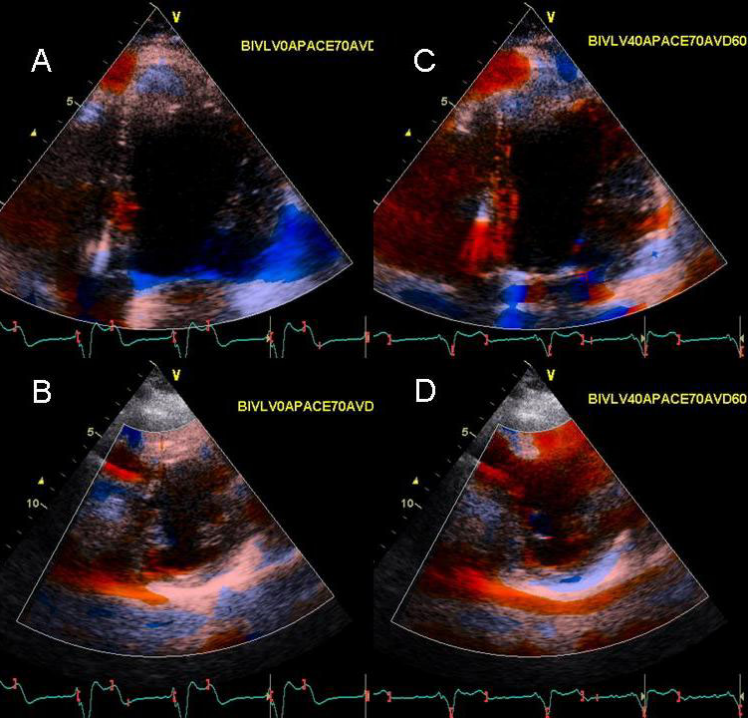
Tissue Doppler strain images in the apical 4 (top panels) and parasternal short axis views (bottom panels) at VV delay of 0 ms (A and B) and during LV pre-excitation of 40 ms (C and D). Positive systolic strain depicted by blue color in the lateral wall, basal interventricular septum and left ventricular apex is shown in A. LV pre-excitation of 40 ms abolished positive strain in the interventricular septum, caused marked reduction of positive strain in the lateral wall and left ventricular apex (C) and improved radial myocardial deformation (negative systolic strain depicted as pink and red myocardial color) in all myocardial segments (D) as well as systolic deformation in right ventricular apex and free wall (C).
